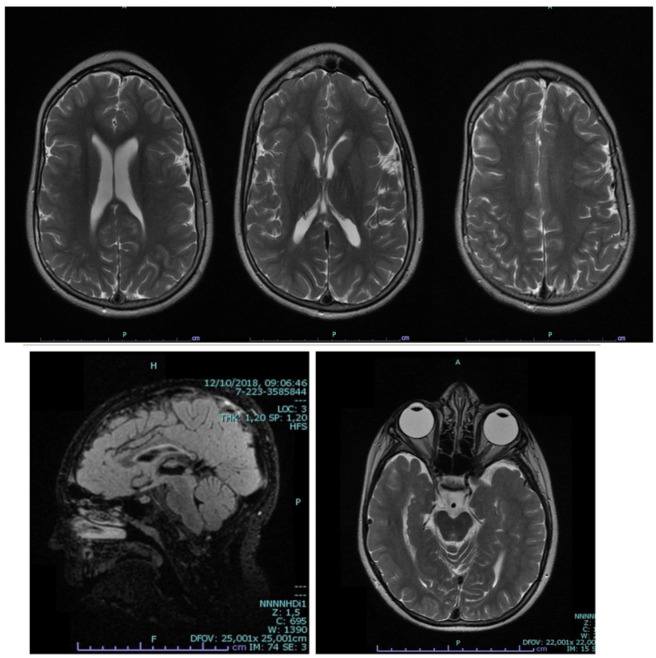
MRI of the reported patient showing the shape of the head, thinning of the corpus callosum, and white matter hypotrophy.
